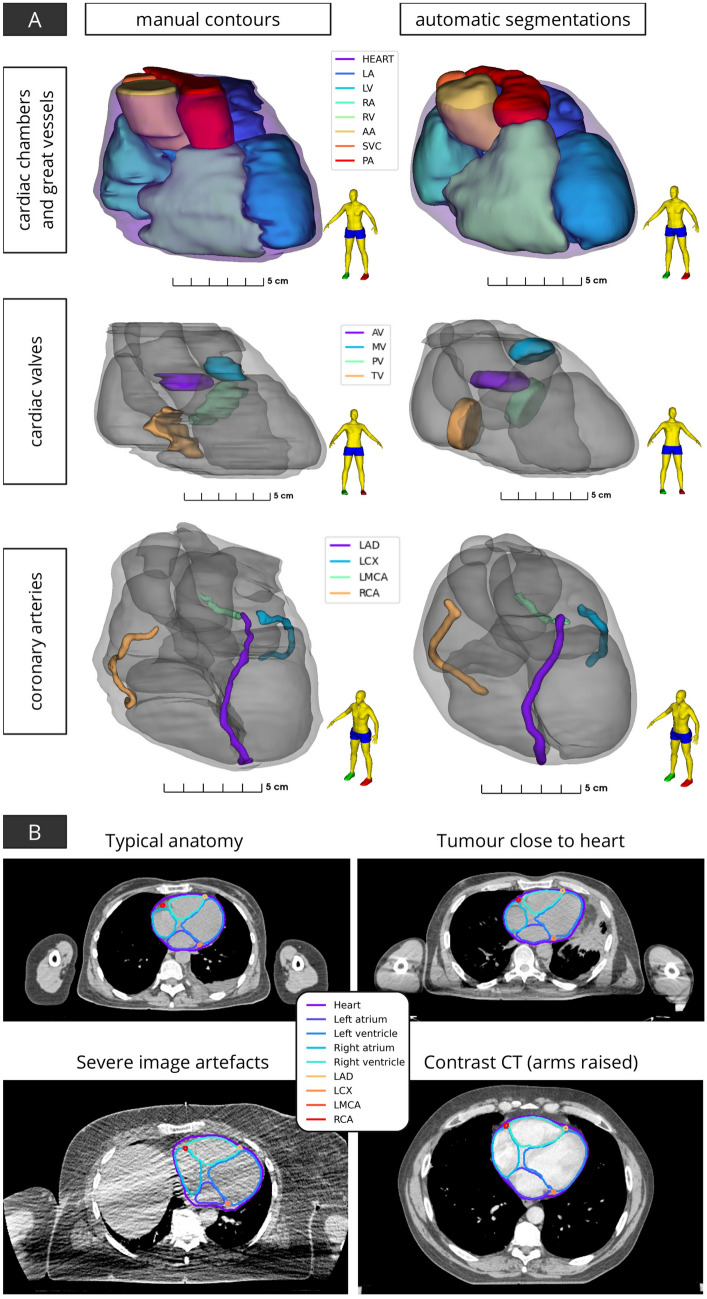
A Surface renderings of manual contours and automatic segmentations for a representative case (median segmentation accuracy). B Axial slices of radiotherapy planning CT scans shown with automatic segmentations for a number of cases with variations in imaging and patient anatomy. Acronyms: LV - left ventricle, RV - right ventricle, LA - left atrium, RA - right atrium, AA - ascending aorta, PA - pulmonary artery, SVC - superior vena cava, AV - aortic valve, PV - pulmonic valve, MV - mitral valve, TV - tricuspid valve, LAD - left anterior descending coronary artery, LCX - left circumflex artery, RCA - right coronary artery, LMCA - left main coronary artery
